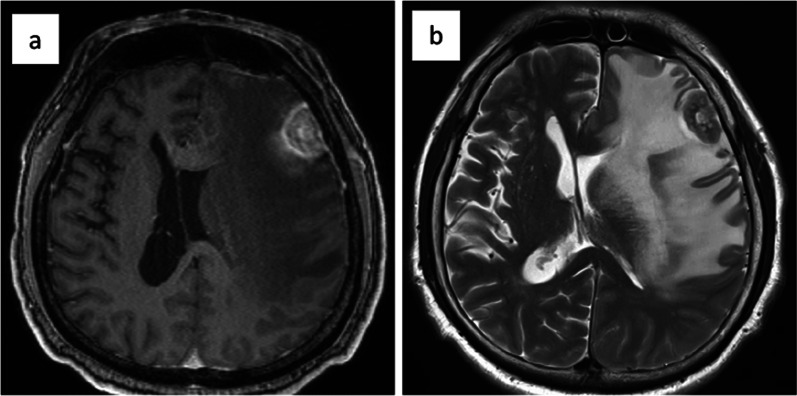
Magnetic resonance imaging of the brain tumor. a T1-weighted image showed a nodular lesion (diameter 13 × 26 mm) near the surface of the left frontal lobe. b T2-weighted image of a high signal area in the surrounding white matter with median deviation
